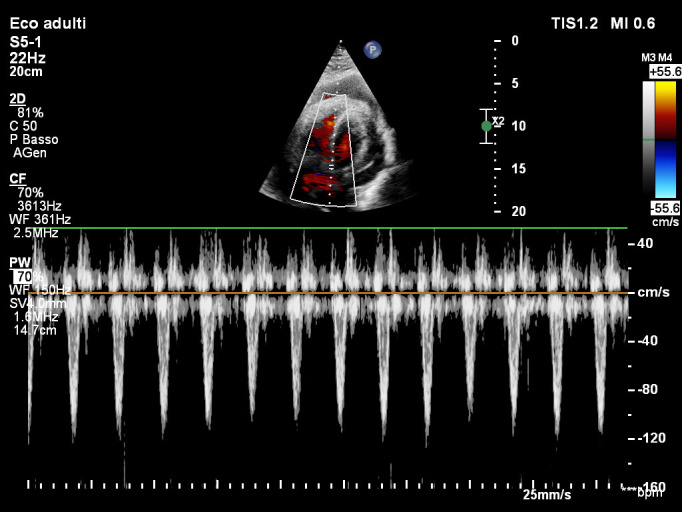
LVOT VTI under positive-pressure ventilation in cardiac tamponade physiology. Pulsed-wave Doppler of the LVOT shows marked respiratory variation in systolic ejection, consistent with exaggerated ventricular interdependence and ventilator-phase–dependent hemodynamic fluctuation. Abbreviations: LVOT, left ventricular outflow tract; VTI, velocity–time integral.
